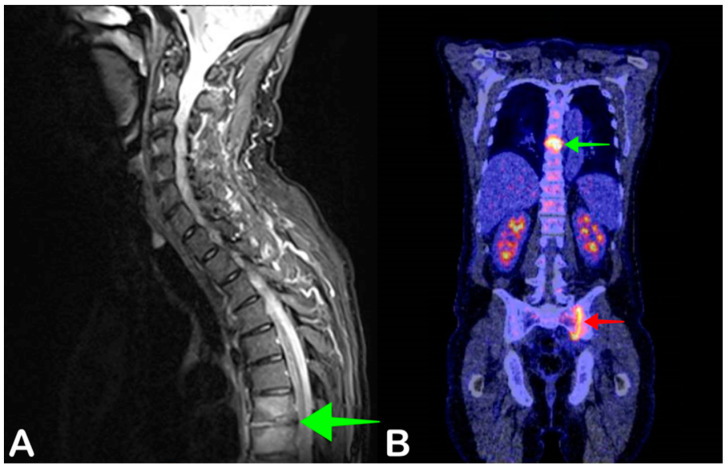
A 63-year-old man presented with fever and back pain. His left upper leg was also painful. Blood cultures were positive for *Staphylococcus aureus.* Sagittal fat-suppressed T2-weighted MRI showed increased signal intensity of thoracic vertebrae seven and eight, and the seventh and eighth intervertebral discs of the thoracic vertebrae–disc complex confirming the spondylodiscitis found on MRI (A, green arrow), but also showed increased FDG uptake at the left sacroiliac joint, indicative of sacroiliitis (B, red arrow). Antibiotic therapy was continued for six weeks after which the patient recovered.
